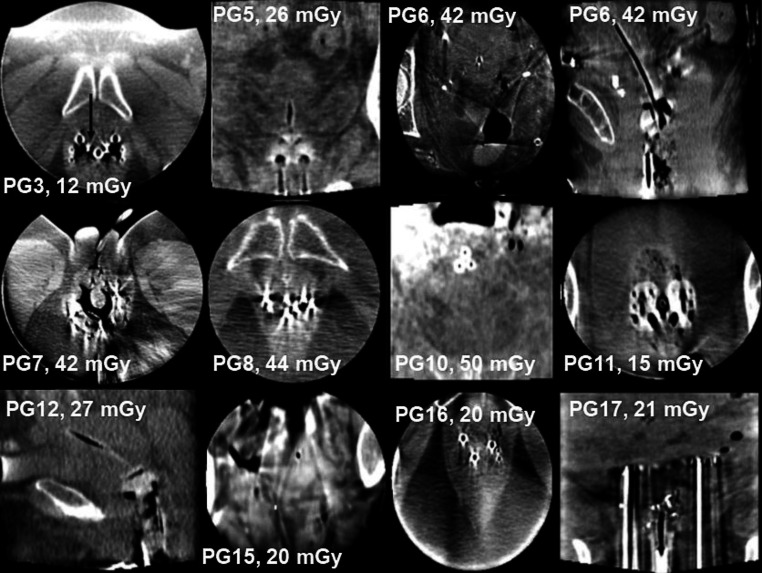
Representative control-CBCTs of gynecologic patients. The applicators were identifiable in each case. For PG3, the applicator–transfer tube assignment was checked by following the inserted X‑ray markers (*black arrow*). However, particularly in sagittal/coronal planes, no clear soft tissue differentiation was feasible in general. Significant artifacts at air–tissue transitions (e.g., PG6, PG10, PG15), directional noise (e.g., PG8, PG11, PG16), and hardening artifacts (e.g., PG3, PG8, PG17) appeared. Images were selected so that each applicator type was represented at least twice, or once if it was used once only. Level: 40 HU, Width: 500 HU
